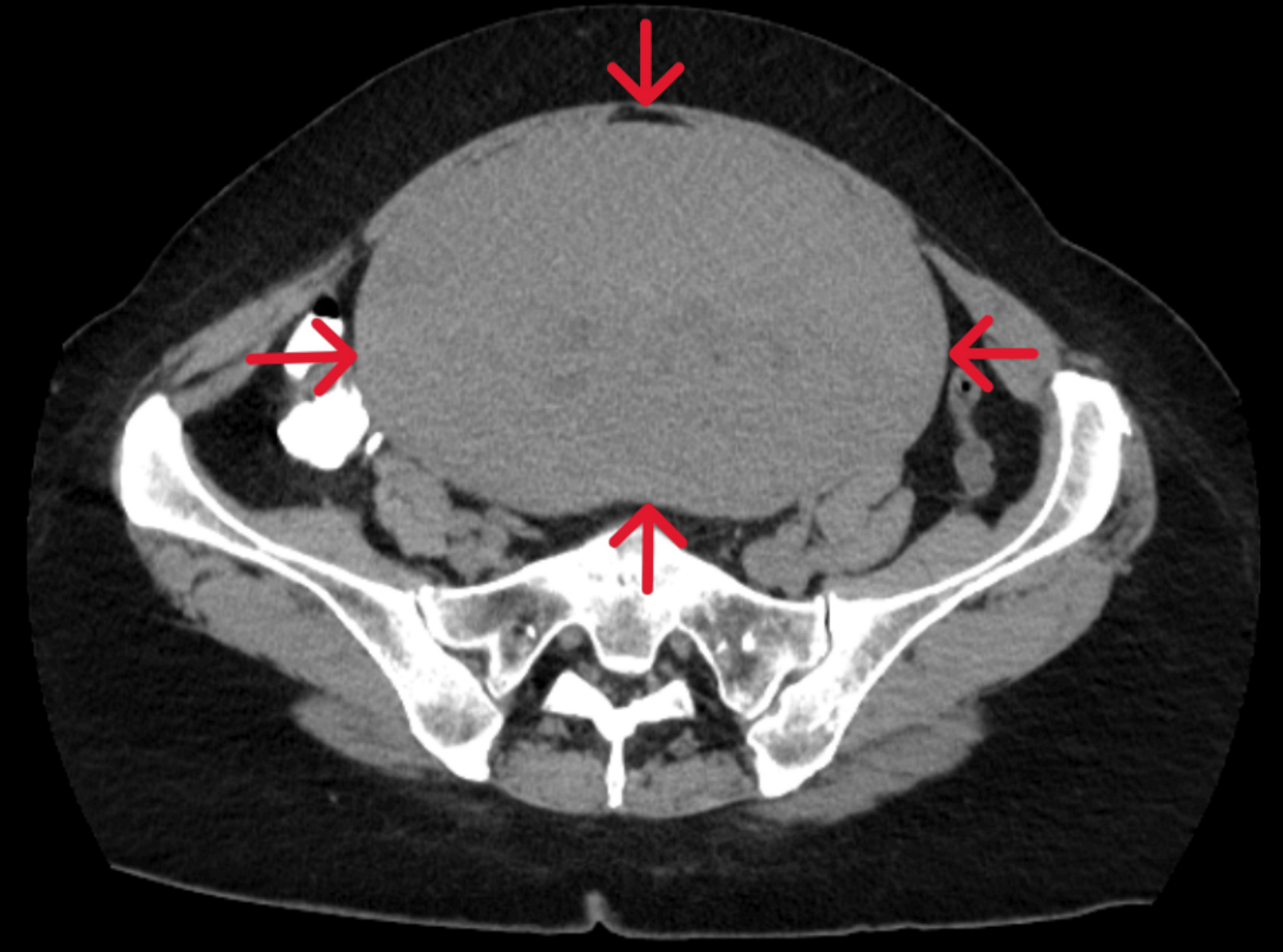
CT imaging of a giant uterine leiomyoma The scan reveals a well-defined pelvic mass of significant size with solid characteristics (red arrows), occupying the majority of the peritoneal cavity.
